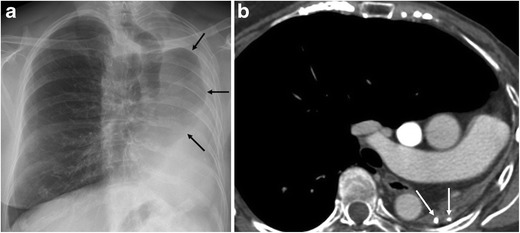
A 68-year-old female with a background of pulmonary TB at age 29 was seen at our hospital for a breast cancer follow-up study. (a) Chest radiography shows marked loss of left lung volume and herniation of the contralateral lung (arrows). (b) Contrast-enhanced multislice CT demonstrates total left lung destruction with no residual cystic bronchiectasis. Calcifications are seen in the remnant lung (arrows), and the contralateral lung is herniated
